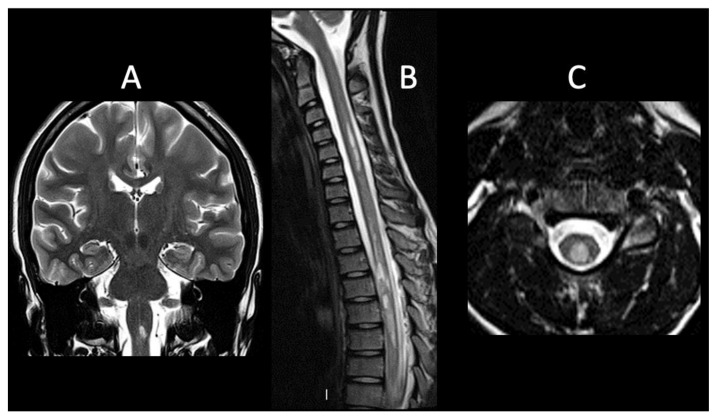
NMOSDs with AQP4-IgG. (A). Coronal T2-weighted image (WI) shows hyperintense lesion in brainstem (case 1), (B). sagittal T2-WI shows multiple and confluent hyperintense lesions in cervical and dorsal spine consistent with LETM (case 2), (C). axial T2-WI shows hyperintense lesion in cervical spine (case 2).
